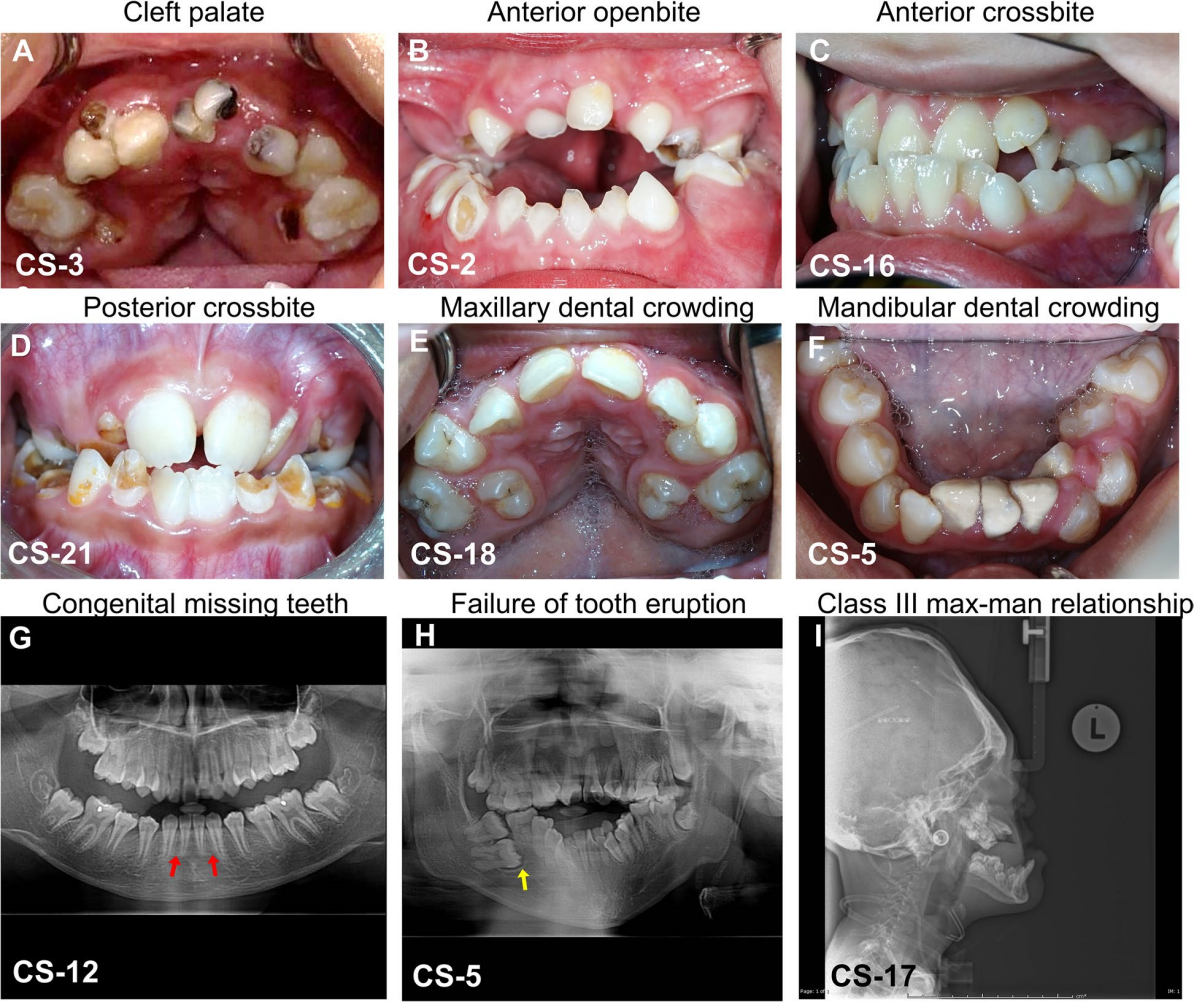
Intraoral features and radiographic images of craniosynostosis (CS) patients. A A 10-year-old boy diagnosed with Apert syndrome (CS-3) showed cleft palate. B A 7-year-old girl with Apert syndrome (CS-2) manifested anterior openbite due to underdeveloped maxilla. C A 10-year-old boy with Crouzon syndrome (CS-16) demonstrated anterior crossbite. D A 6-year-old boy with multiple sutures CS (CS-21) exhibited posterior crossbite. E A 12-year-old boy with Apert syndrome (CS-18) had maxillary dental crowding. F A 15-year-old girl with Apert syndrome (CS-5) manifested mandibular dental crowding. G Panoramic film of A 9-year-old girl with Apert syndrome (CS-2) exhibited congenital missing lower lateral incisor teeth (arrows). H Panoramic film of a 15-year-old girl with Apert syndrome (CS-5) showed failure of tooth eruption (arrow). I Lateral cephalogram of a 10-year-old girl with Crouzon syndrome (CS-17) demonstrated Class III maxillomandibular relationship
